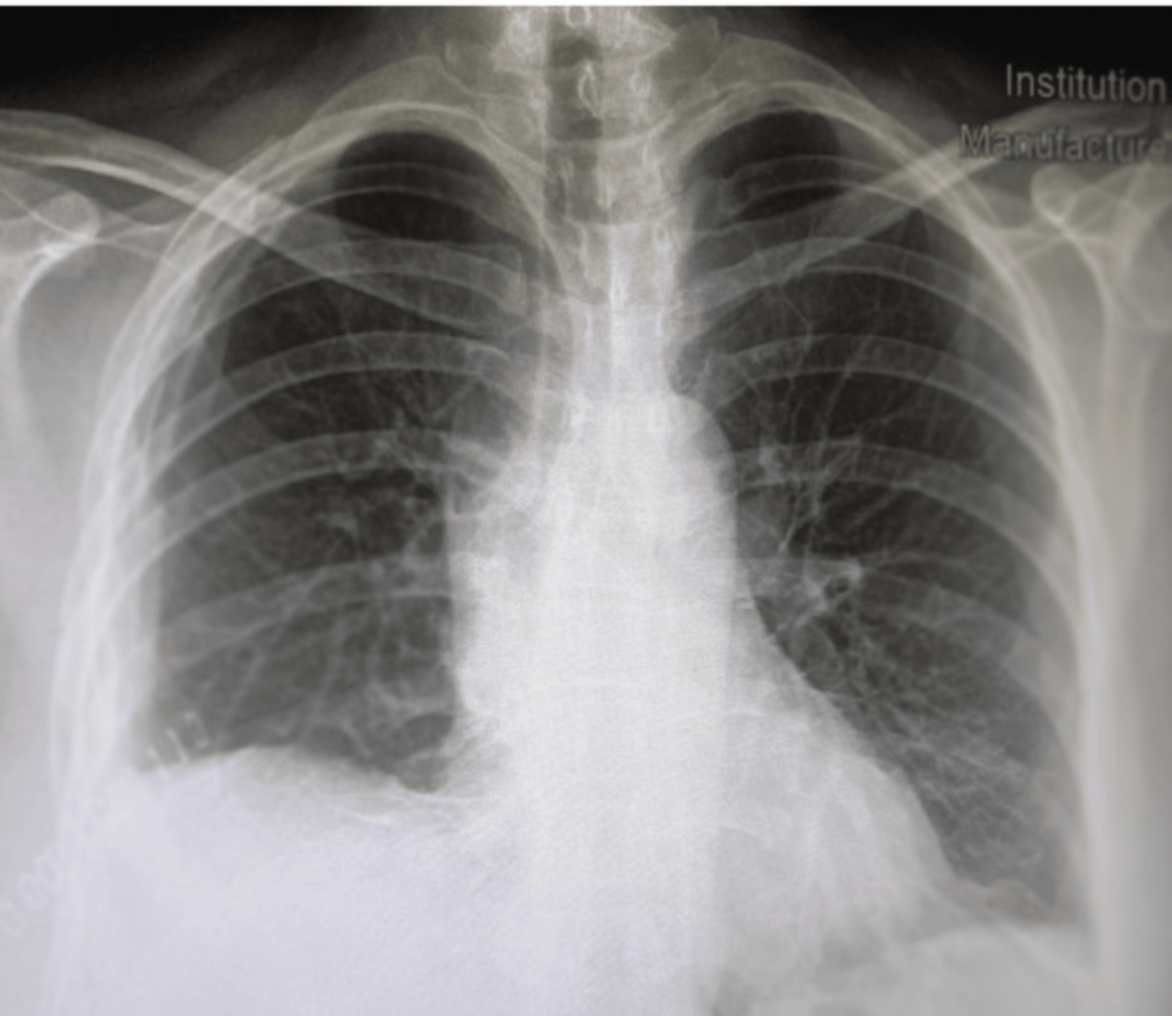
Postoperative imaging A chest radiograph from postoperative day 2 demonstrating the satisfactory position of the Bülau drainage tube in the right hemithorax.
